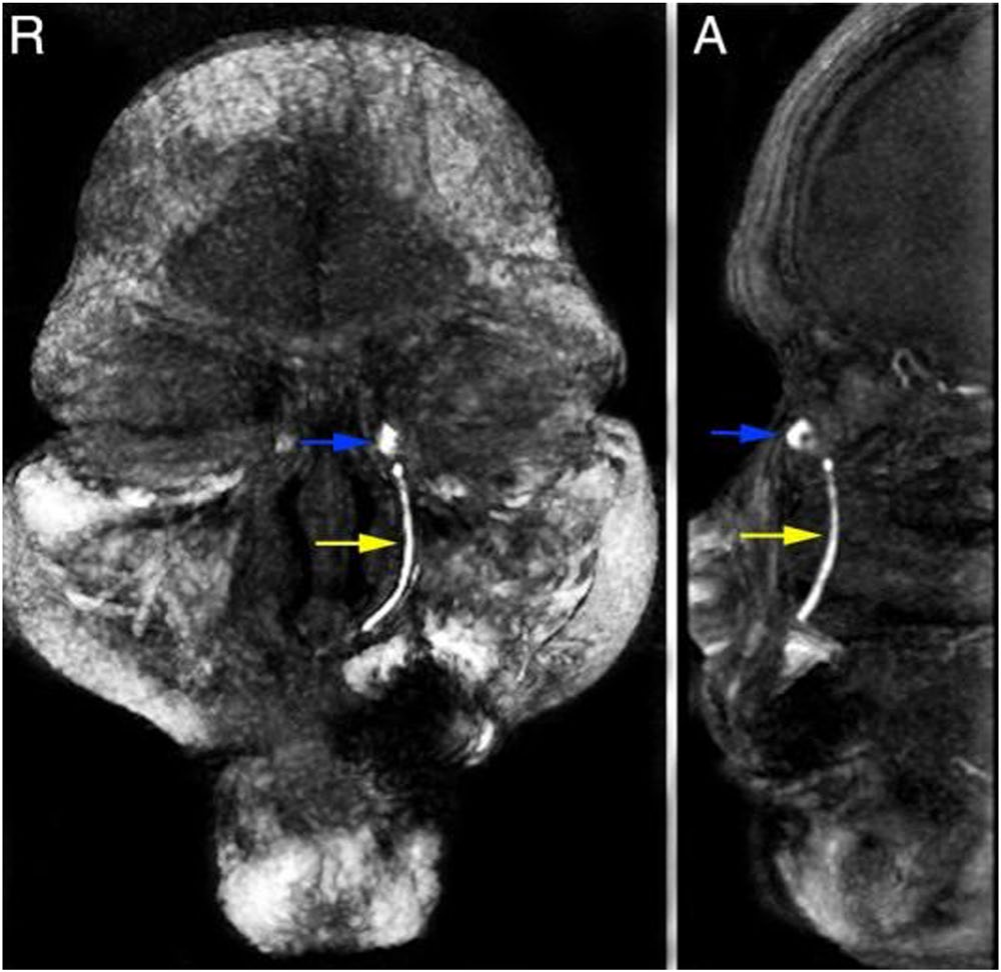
The MRI after RSHSI. The bule arrow represents the lacrimal sac. The yellow arrow marks the Spherical Headed Silicone Tube. R, Right; A, Ahead.
